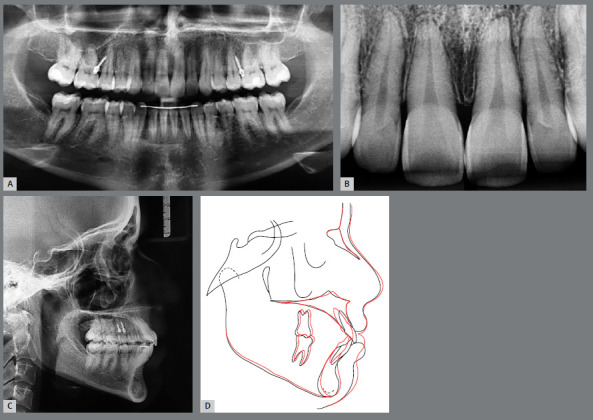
Final panoramic radiograph (A), periapical radiograph (B), and lateral cephalometric radiograph(C). Initial and final tracings superimposition (D).
